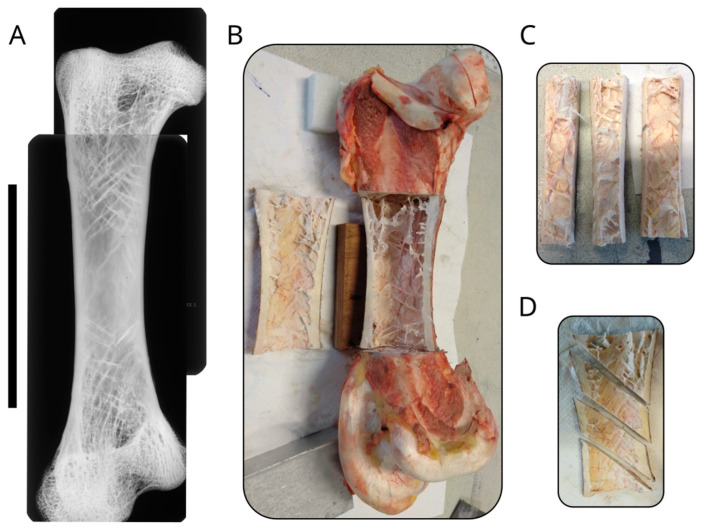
Structure of the ostrich femur. (A) Image composed of multiple radiographic images of an ostrich femur, showing the distribution of the trabeculae. The scale bar is equal to 10 cm. (B) Photo of the sectioned femur showing the marrow cavity with the oriented trabeculae. (C) Photo of the samples oriented alongside the long axis of the femur. (D) Photo of the samples oriented at 45° with respect to the long axis of the bone.
